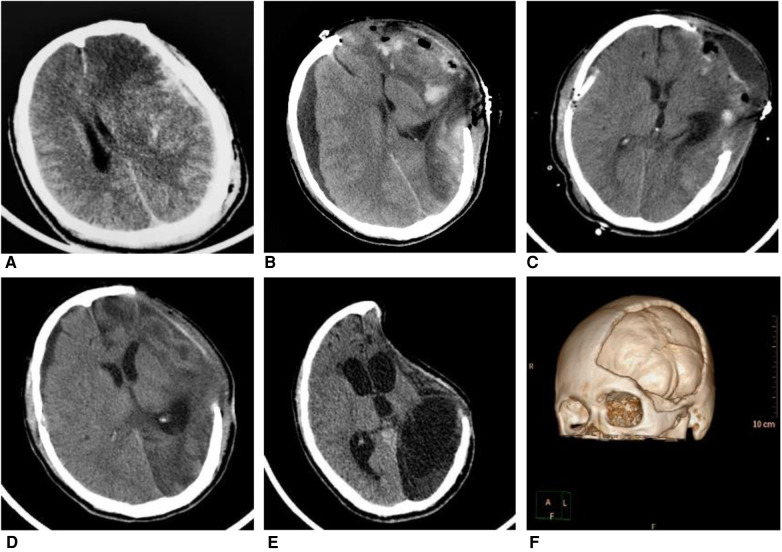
A 31-year-old man fell from a height of three meters after electric shock, whose GCS is 4 points at admission. Patient received decompressive craniectomy (DC) and absorbable dura repairment. (A) Computed tomography (CT) image at admission showed an acute subdural hematoma on the left side. (B) CT at 11 days after DC showed subdural hygroma on the right side and herniation of brain tissue on the left side. (C) Patient received burr hole irrigation and drainage 13 days after DC, postoperative CT showed the reduction of subdural hygroma. (D) CT at one month after DC showed shrinkage of left-sided brain tissue (E) Six months after DC, CT indicated the syndrome of the trephined (F) 3D reconstruction of the CT scan of this patient after DC.
